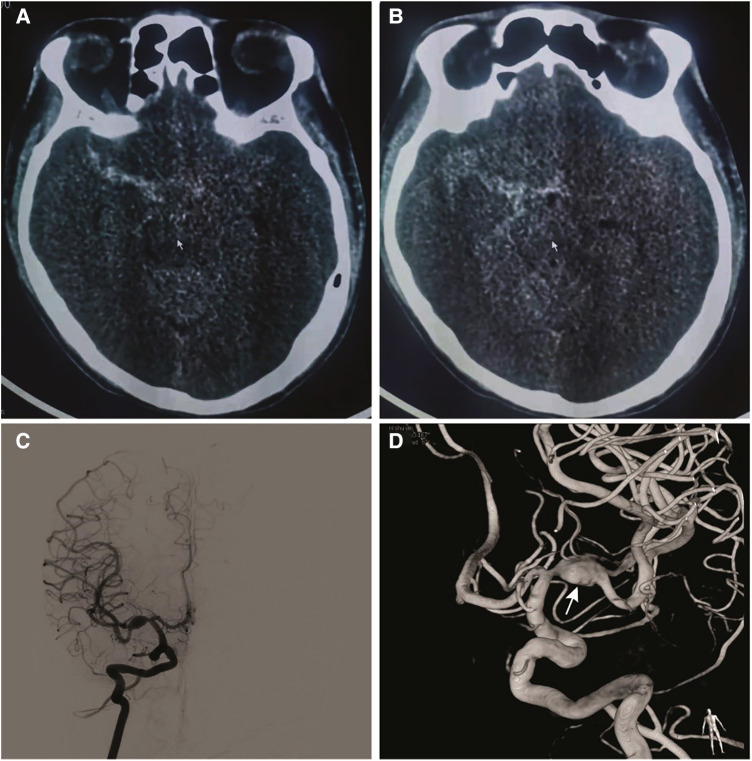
(A) and (B) computed tomography of the head demonstrating subarachnoid hemorrhage. (C) Anterior-posterior the right internal carotid artery and (D) 3D rotational angiogram demonstrating a fusiform aneurysm of the M1 segment of the right MCA measuring 10.11 × 6.47 mm. Multiple ascuses (arrow) were visible in the inferior wall of aneurysm.
